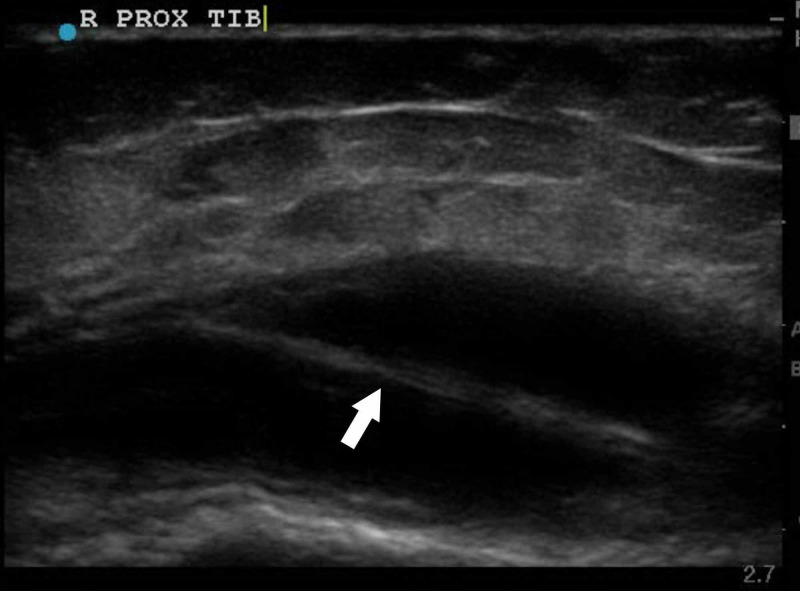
Longitudinal view of the proximal tibia demonstrating fluid collection, with arrow showing large septation (later demonstrated to be fascia) on bedside ultrasound.
